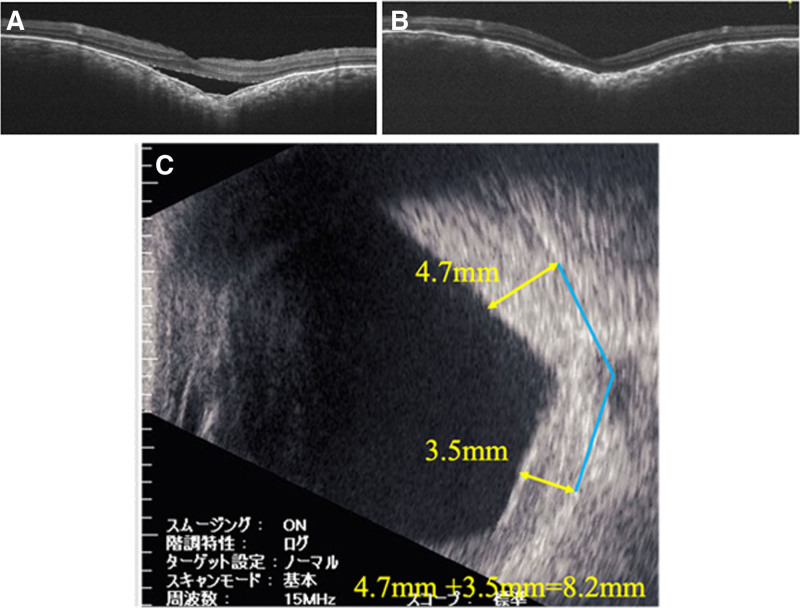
(A) OCT when retinal detachment recurred in the right eye in April 2019. (B) OCT when retinal detachment improved in the right eye in May 2020. (C) B-mode ophthalmic ultrasound in May 2020 after recurrent retinal detachment and improvement in the right eye. The height of the hemangioma was 8.2 mm (4.7 + 3.5 mm). OCT = optical coherence tomography.
